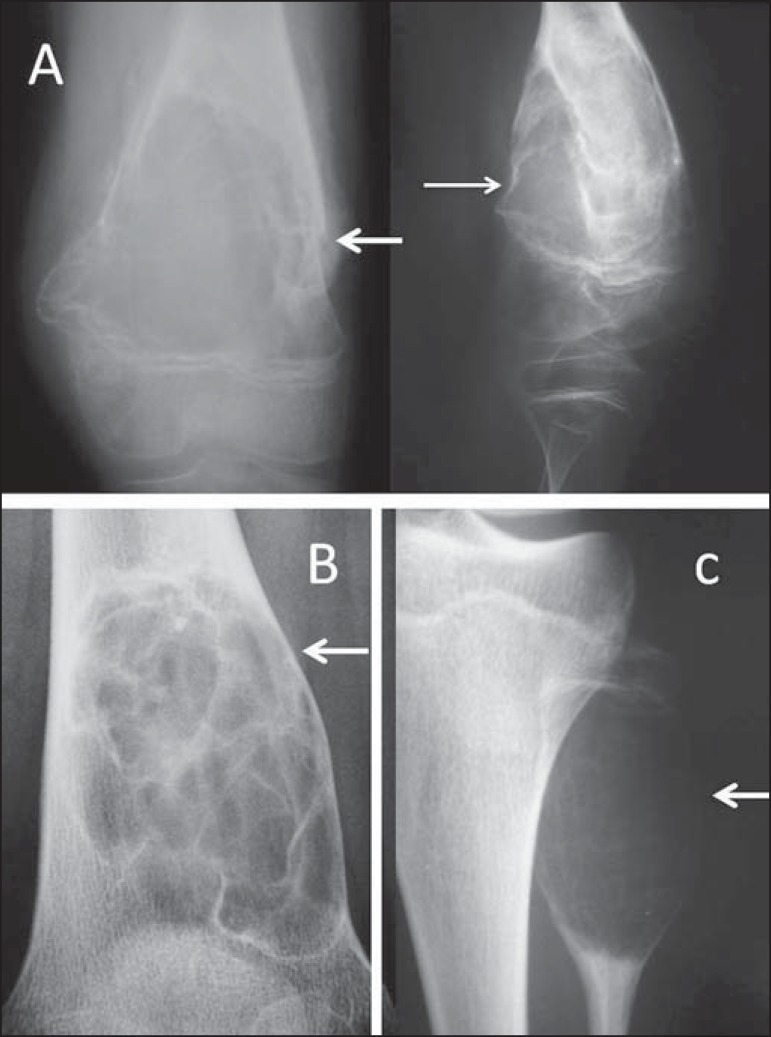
A: Conventional anteroposterior and lateral radiography
